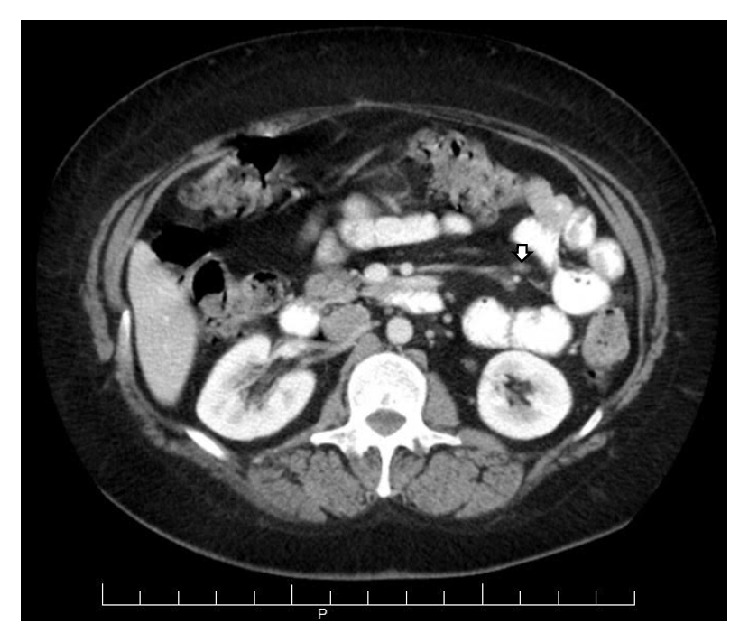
Axial CT image after therapeutic intervention reveals response to therapy with a decrease in the size of the mesenteric lymph node (arrow) and resolution of mesenteric fat stranding.
